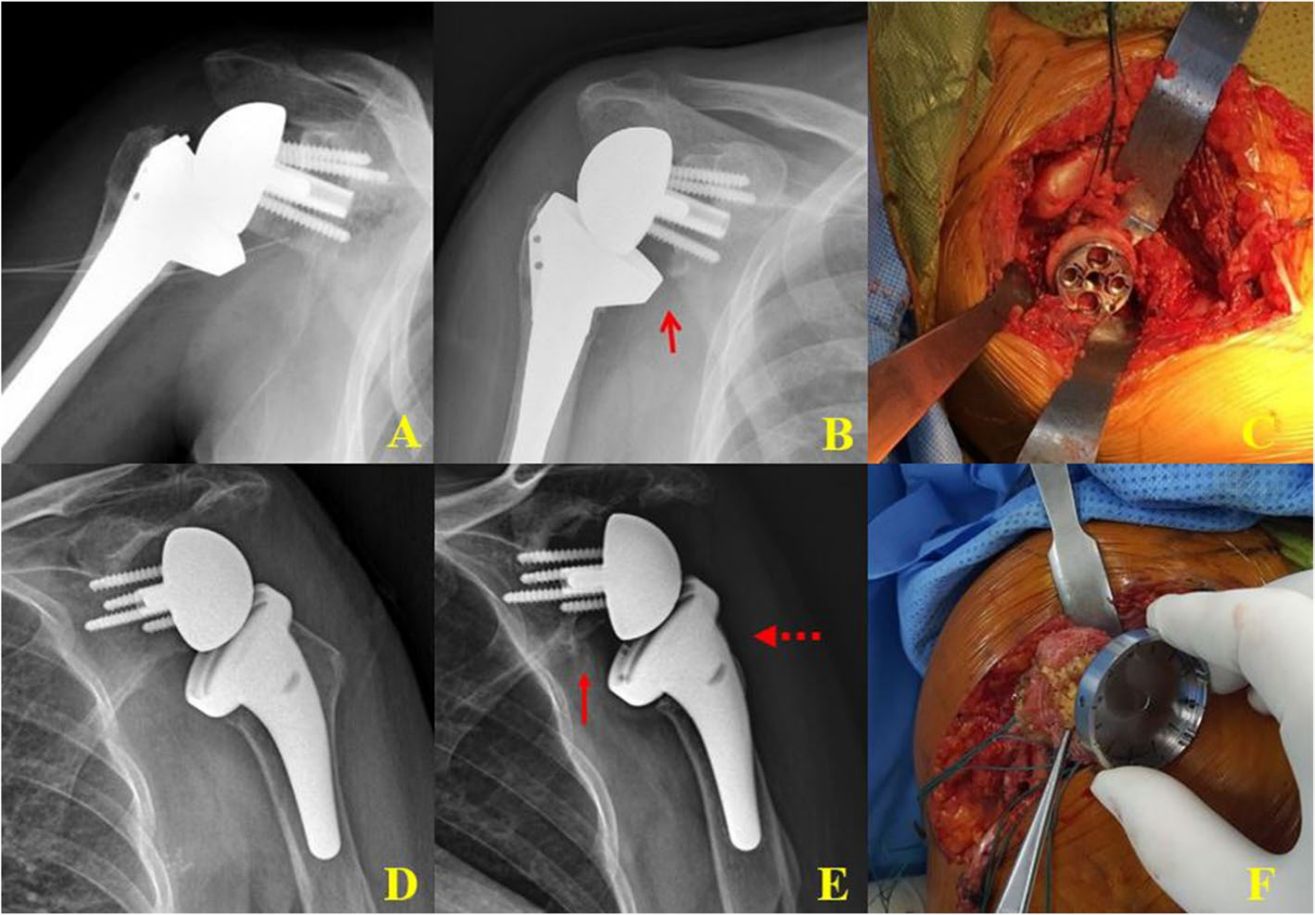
Comparison of glenoid lateralization (bony increased offset) reverse total shoulder arthroplasty) and humeral lateralized (cementless, curved, short-stem) reverse shoulder arthroplasty. Radiologic findings show the inserted bone graft on the glenoid in the glenoid lateralization design (A). A 4-year follow-up X-ray showing Grade 3 scapular notching on the scapular neck (B). Intraoperative photo showing that the autograft was well attached to the glenoid long post baseplate and inserted into the native glenoid (C). Radiologic findings showing the curved, short-stem insertion in the humeral lateralization design (D). A 3-year follow-up X-ray showing Grade 3 scapular notching on the scapular neck (E). Intraoperative photo showing that the chip bone autograft was inserted into the proximal humerus before stem insertion to prevent early humeral stem loosening (F)
